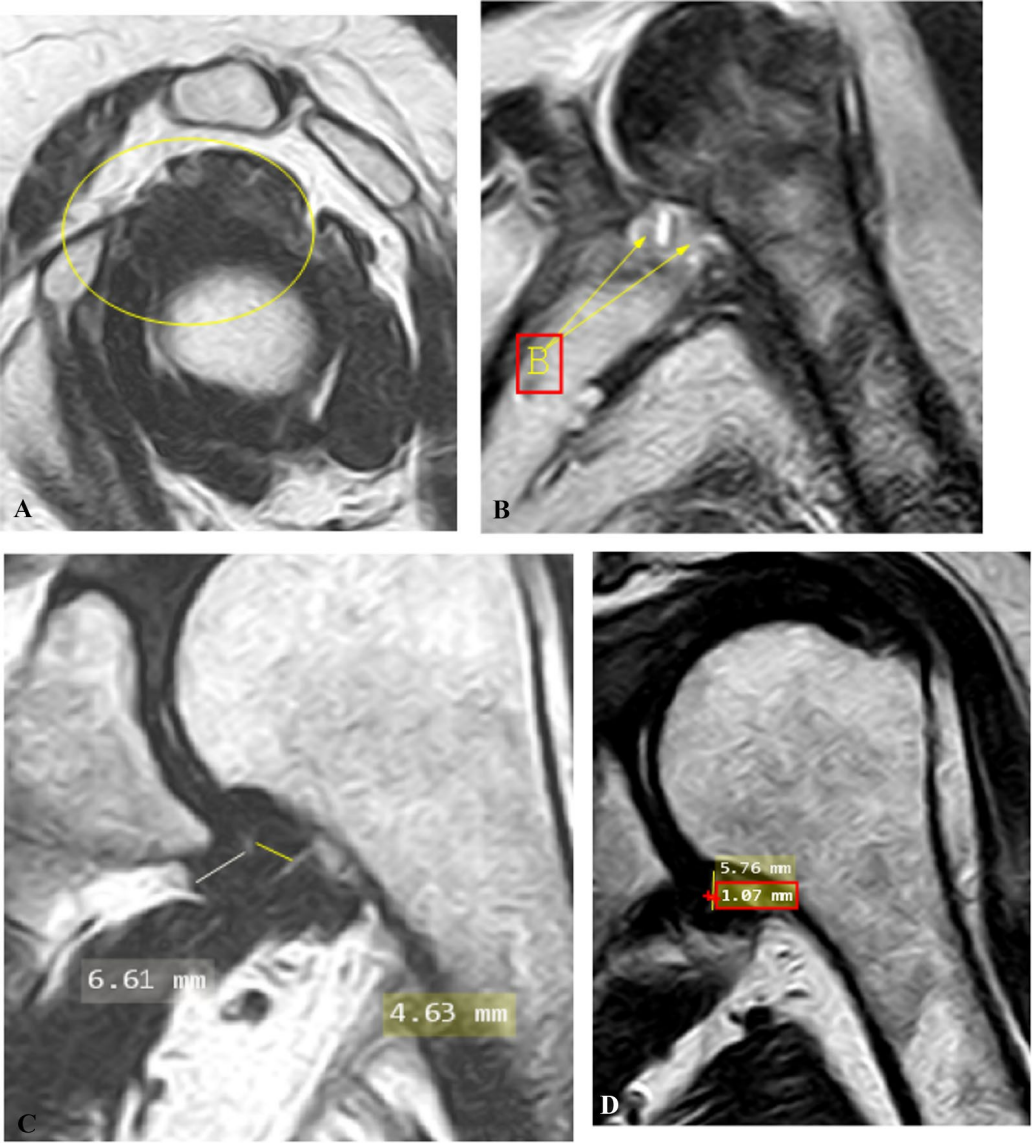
A Oblique sagittal T2-weighted MR image shows complete obliteration of subcoracoid fat triangle with no observed fat planes or coracohumeral ligament (encircled area). B Oblique coronal fat-suppressed PD-weighted MR image shows edema at glenoid and humeral portions of axillary recess (arrows). C Oblique coronal T2-weighted MR image shows measurement of thickest portion of joint capsule in humeral and glenoid portions of axillary recess. D Oblique coronal T2-weight MR image shows measurement of maximal height and width of axillary recess
